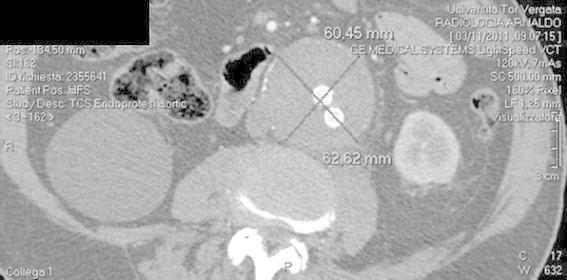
CT scan performed after EVAR procedure. The aneurysm was >6 cm in diameter
